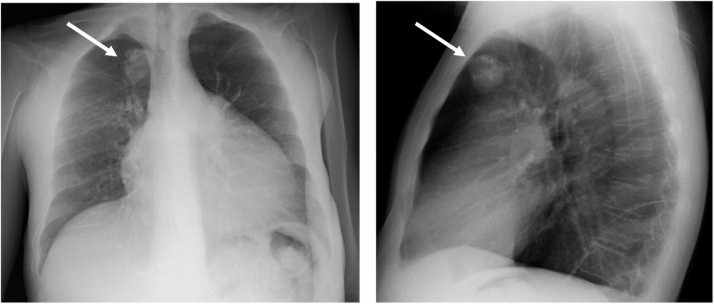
The chest radiograph showed one round calcified tumor (white arrow) in the right lung.•Gastric tumor of 13.5cm × 7.5cm with soft tissue density, extending into pancreas, without infiltration (Fig. 3).
